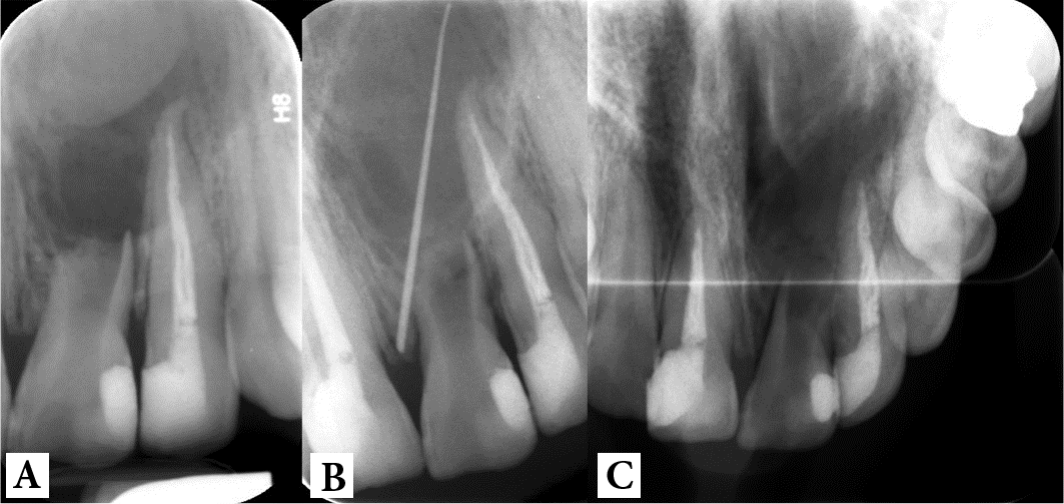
Preoperative radiographs: *A) *periapical;* B) * tracing with gutta-percha and* C) *occlusal view
